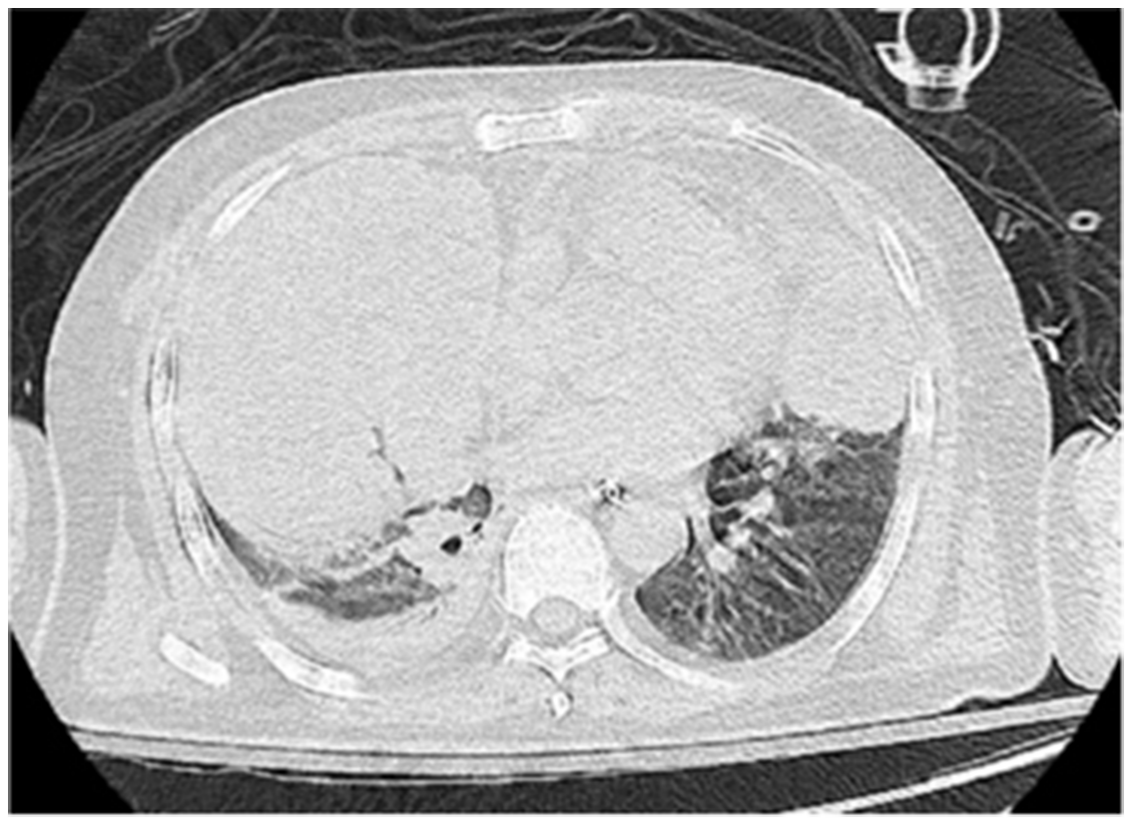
Lung CT scan on the fourth day after weaning from ECMO.
